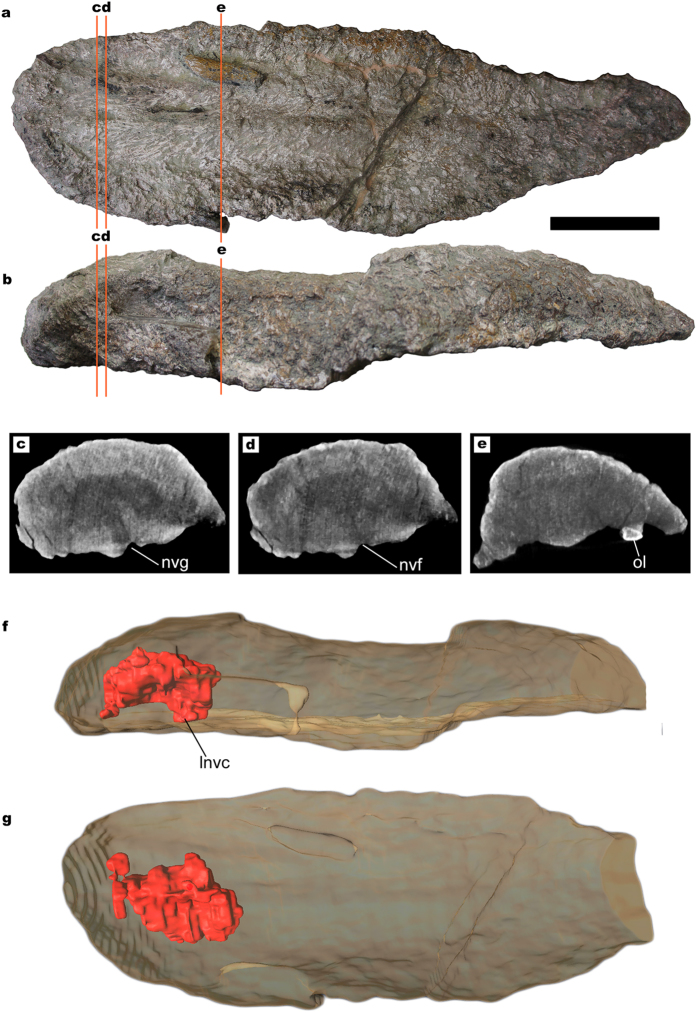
Titanosaur osteoderm with small internal cavities. (a) HUE-00950 in deep view. (b) HUE-00950 in lateral view. (c–e) CT-scan slices at different positions, where the lower density area and the neuro-vascular canal can be observed. (f) 3D model of HUE-00950 in lateral view, obtained from the CT-scan slices, with the void and canal in red. (g) 3D model of HUE-00950 in deep view. Scale = 100 mm. lnvc = longitudinal neurovascular canal. nvf = neurovascular foramen. nvg: neurovascular groove. ol = ossified ligament.
